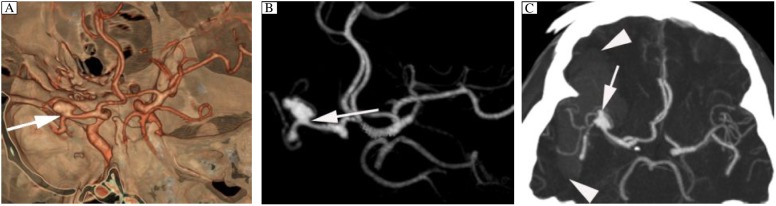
Right middle cerebral artery aneurysm in a 58-year-old man with SAH and intracranial hematoma (IH). A: VR image from CTA clearly display the relationship of the aneurysm to bone structures, adjacent branch vessels, and aneurysmal neck (arrow). B: MIP image from CTA clearly demonstrates the relationship of the aneurysm (arrow). C: Thin-MIP image from CTA clearly demonstrates the relationship of the aneurysm to IH (arrowhead), and the ruptured aneurysm has a small “nipple” (arrow). CTA: computed tomography angiography; MIP: maxium intensity projection; SAH: subarachnoid hemorrhage; VR: volume rendering.
